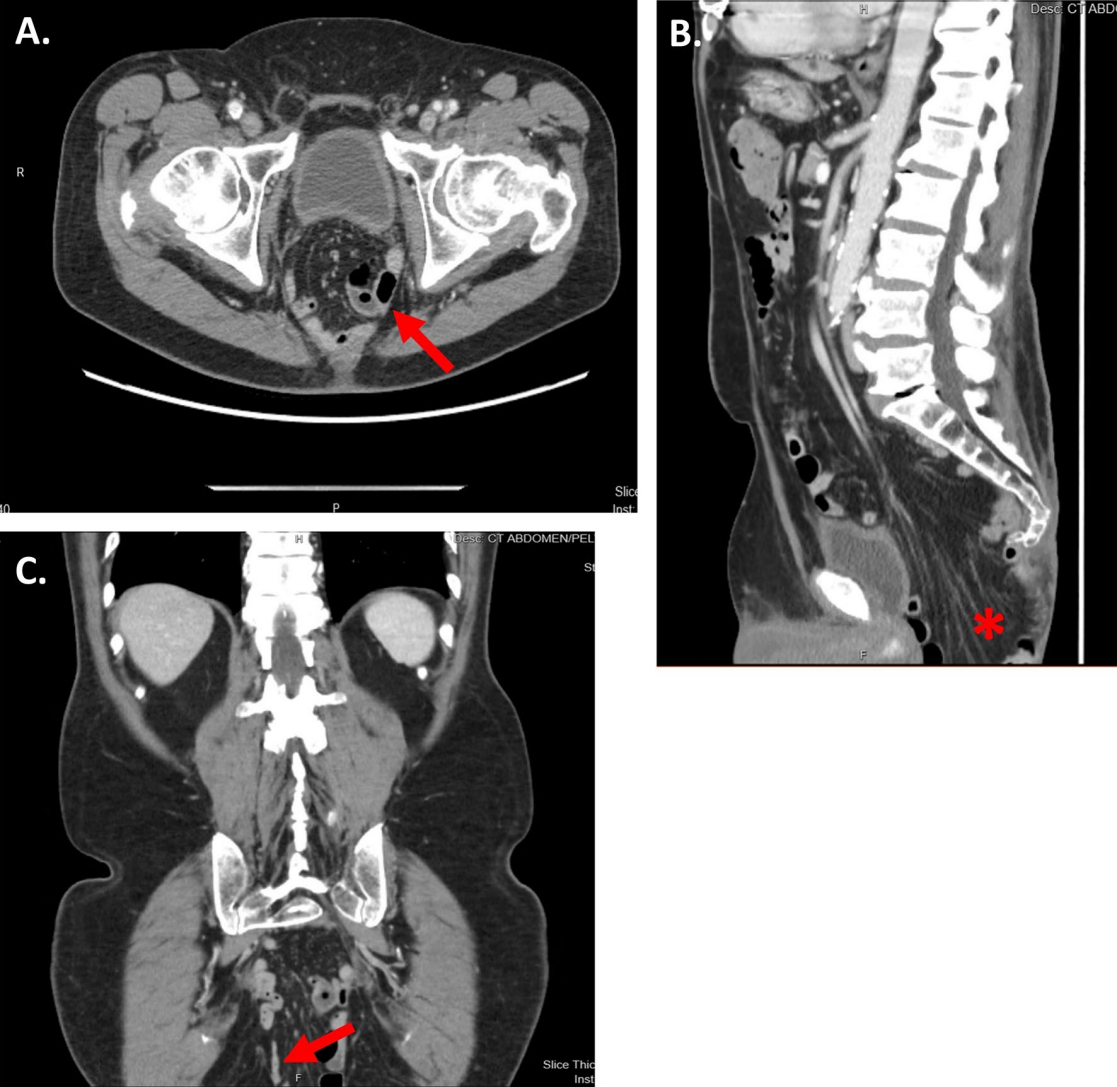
Pre-operative CT abdomen/pelvis showing the hernia defect (red arrow and star) in the A Axial view, B Sagittal view, and C Coronal view (Color figure online)
